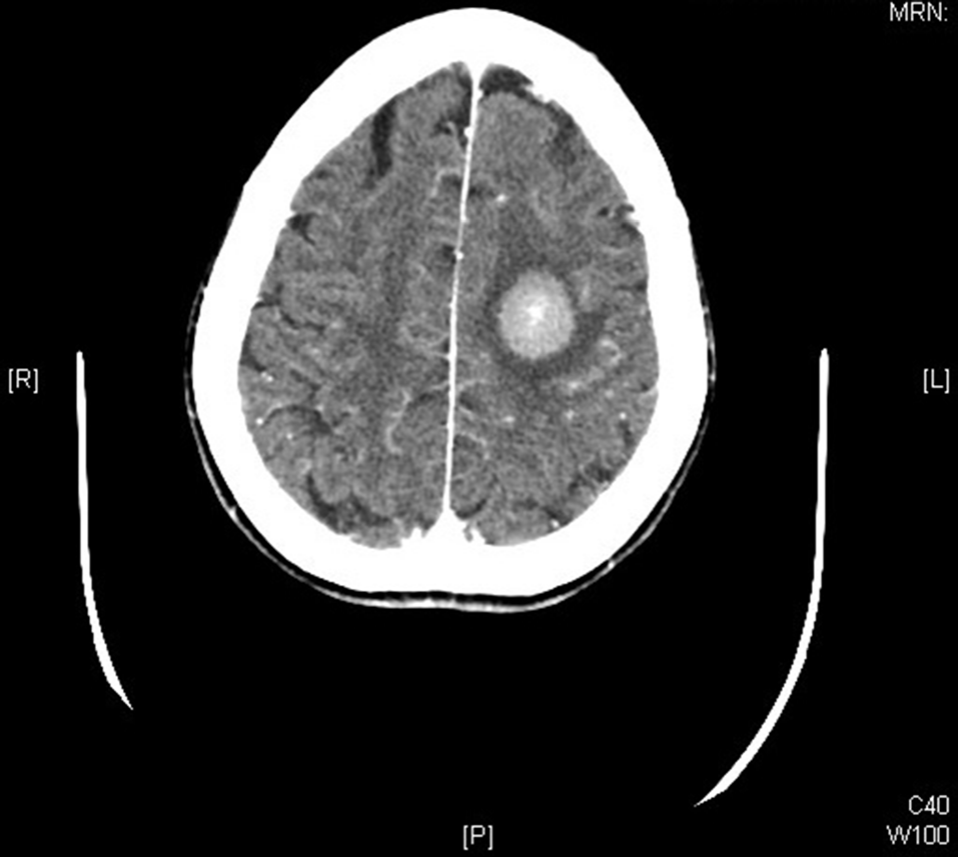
CT angiogram demonstrating intraparenchymal hemorrhage in the left parietal lobe
